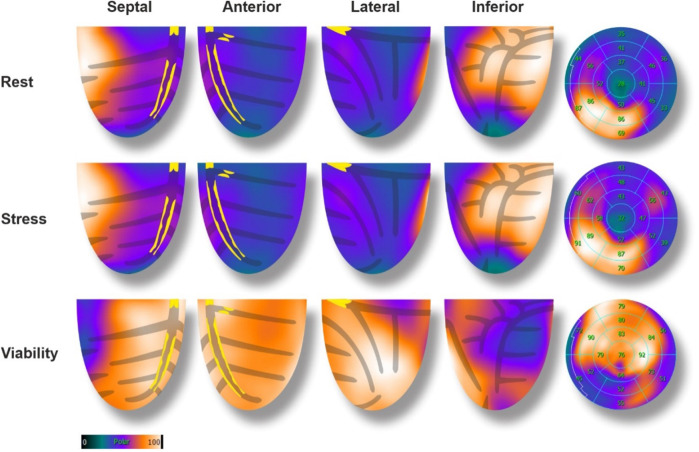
Baseline 82-rubidium PET-CT scan with corresponding glucose metabolism (18F-FDG), and coronary lesions. In perfusion images, a large (70%), severe, anterior, lateral, and apical defect was noted with no reversibility. The 18F-FDG showed enhanced glucose metabolism in the hypo-perfused areas (mirror image), denoting a hibernating state of the myocardial cells.
